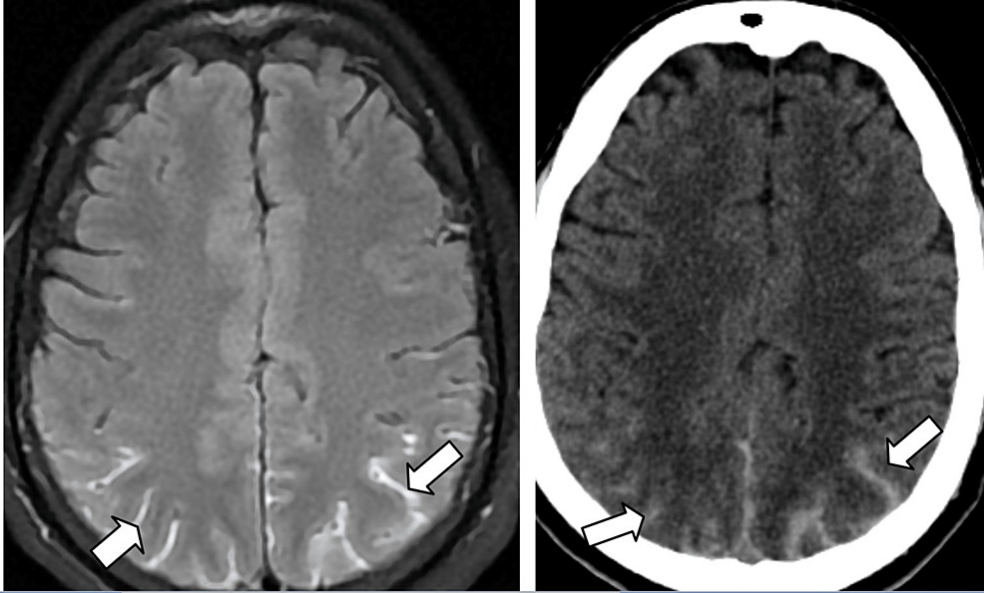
The MRI and CT images demonstrating subarachnoid hemorrhage in bilateral high parietal lobes (arrows) and also in occipital lobes with subtle dependent hemorrhage in bilateral occipital horns of the lateral ventricle (not shown here). A: Axial fluid-attenuated inversion recovery image showing sulcal hyperintensities in the bilateral high parietal lobes (arrows) B: Plain CT image showing mild subarachnoid hemorrhage in the bilateral high parietal lobes in the same patient (arrows)
